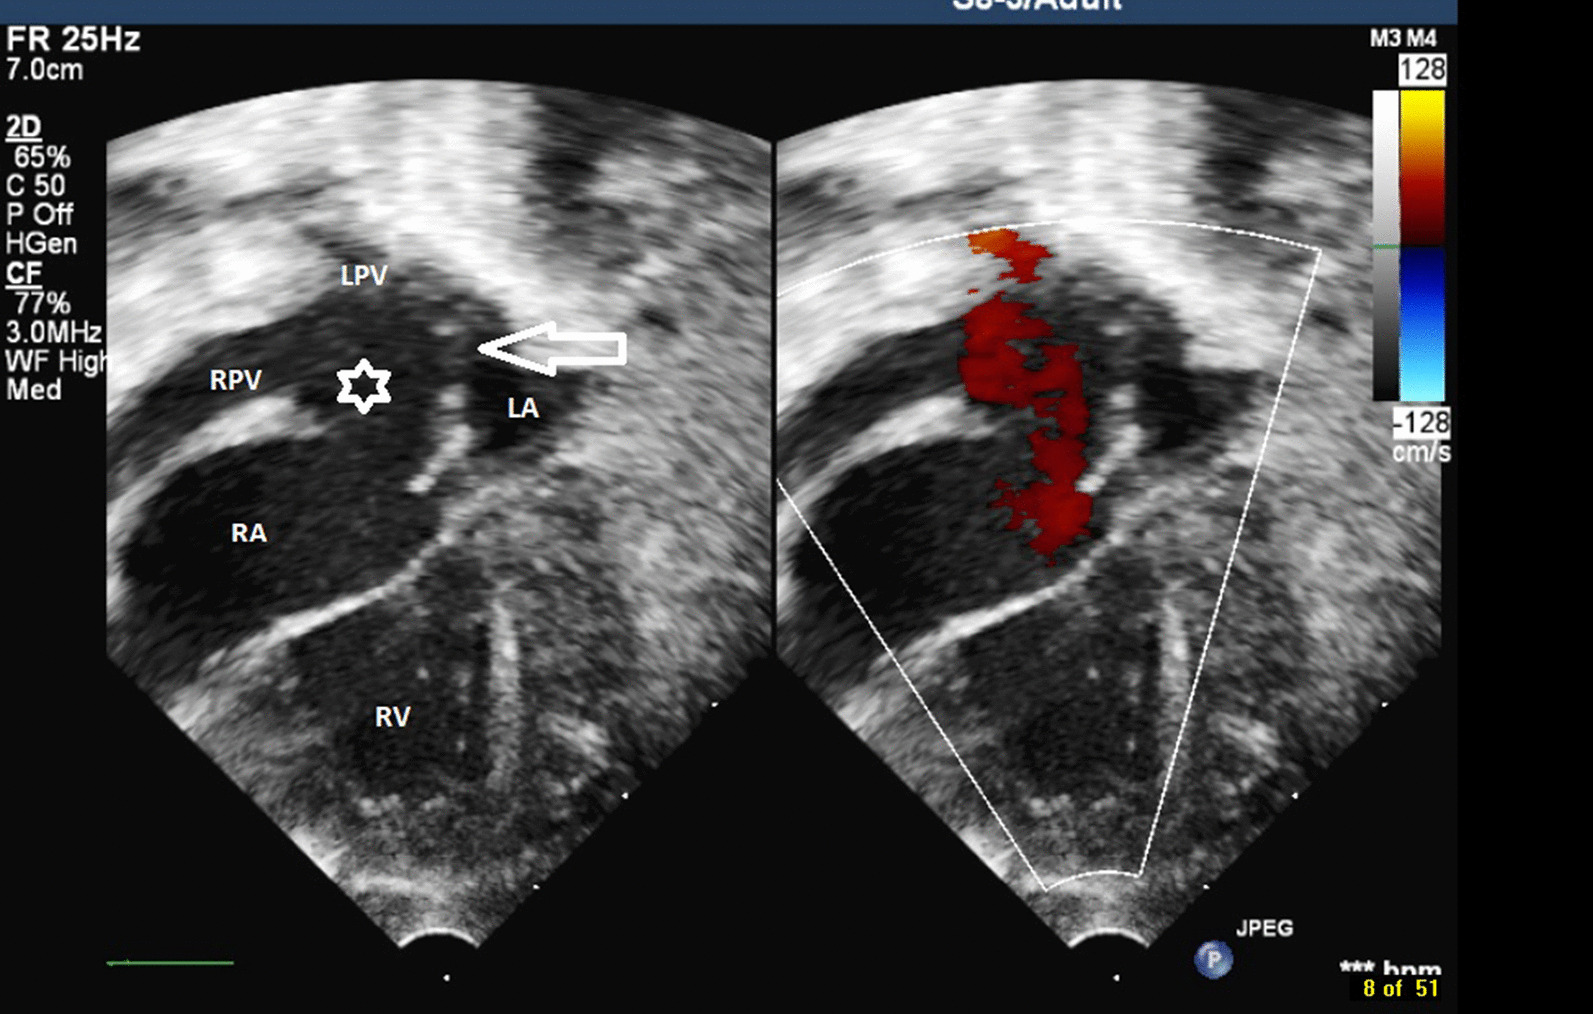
Preoperative 2-D echocardiogram including colour Doppler showing TAPVR into the RA (RPV—right pulmonary veins, LPV—left pulmonary veins, RA—right atrium, LA—left atrium, RV—right ventricle, white star—TAPVR to the RA, White arrow—inter atrial communication)
